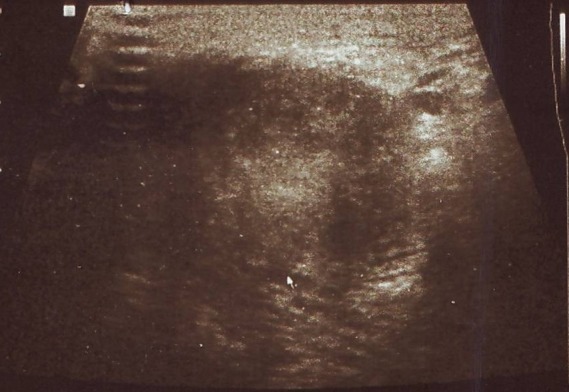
Vulva sonography shows hypoechoic mass 2.9×2.5×2.1 cm.
